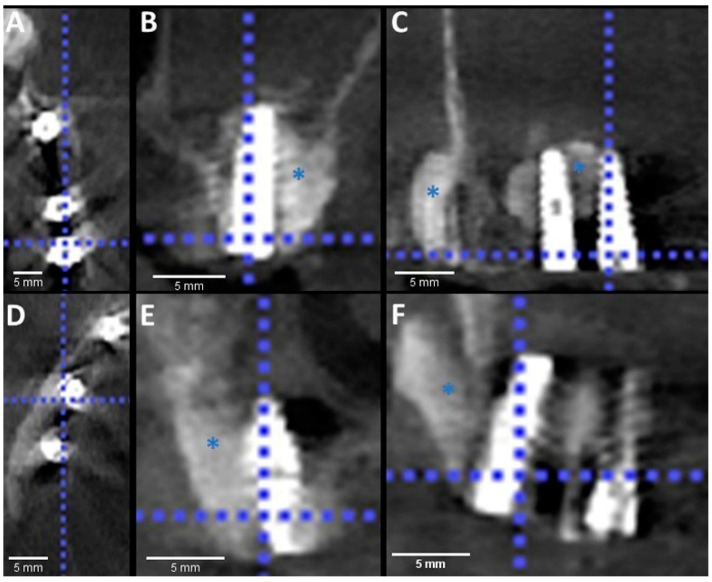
Postoperative CBCT imaging showed good integration between the cement, implant and bone. Paste-CPC is seen around the implants within the bone defect. Zoom enhanced axial (A,D), coronal (B,E) and sagittal (C,F) views, on the left side (upper panel) and the right side (lower panel). Blue asterisks mark the cement.
